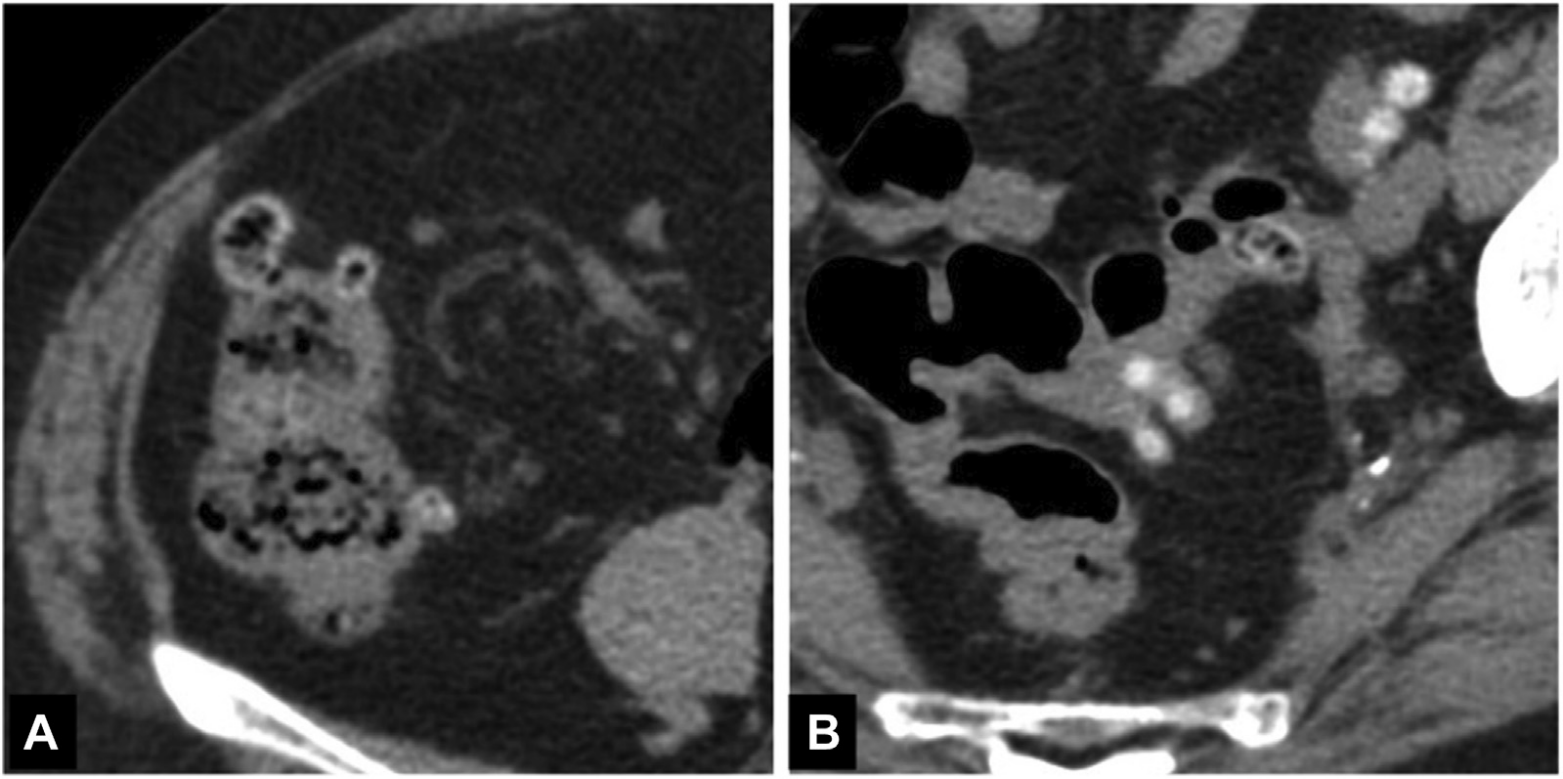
Plain computed tomography scan images. A, Diverticula in the ascending colon. B, Diverticula in the sigmoid colon.
